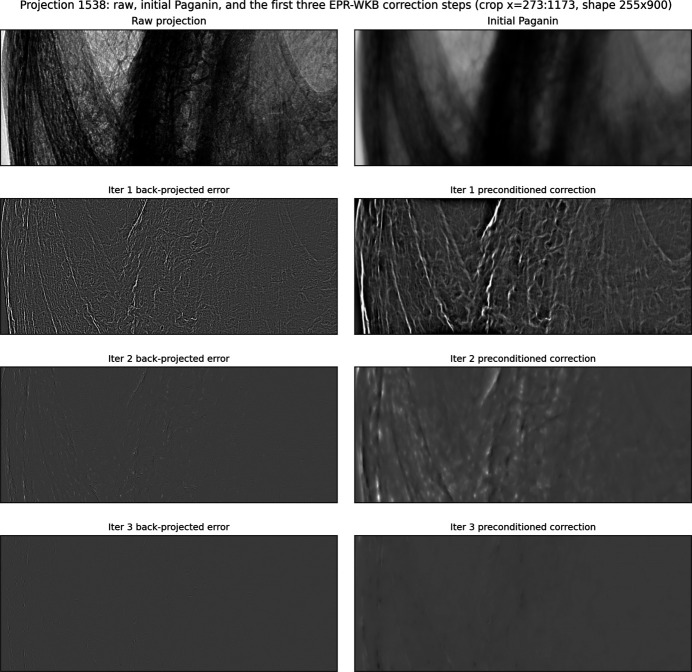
Evolution of the fast non-local phase retrieval in the projection domain for the same representative radiograph. The first row shows the raw projection and the initial Paganin estimate. The following rows show the back-projected residual and the preconditioned correction after iterations 1, 2 and 3. For each quantity type, the same grayscale range is used across iterations, making the rapid decrease of the correction amplitudes directly visible.
